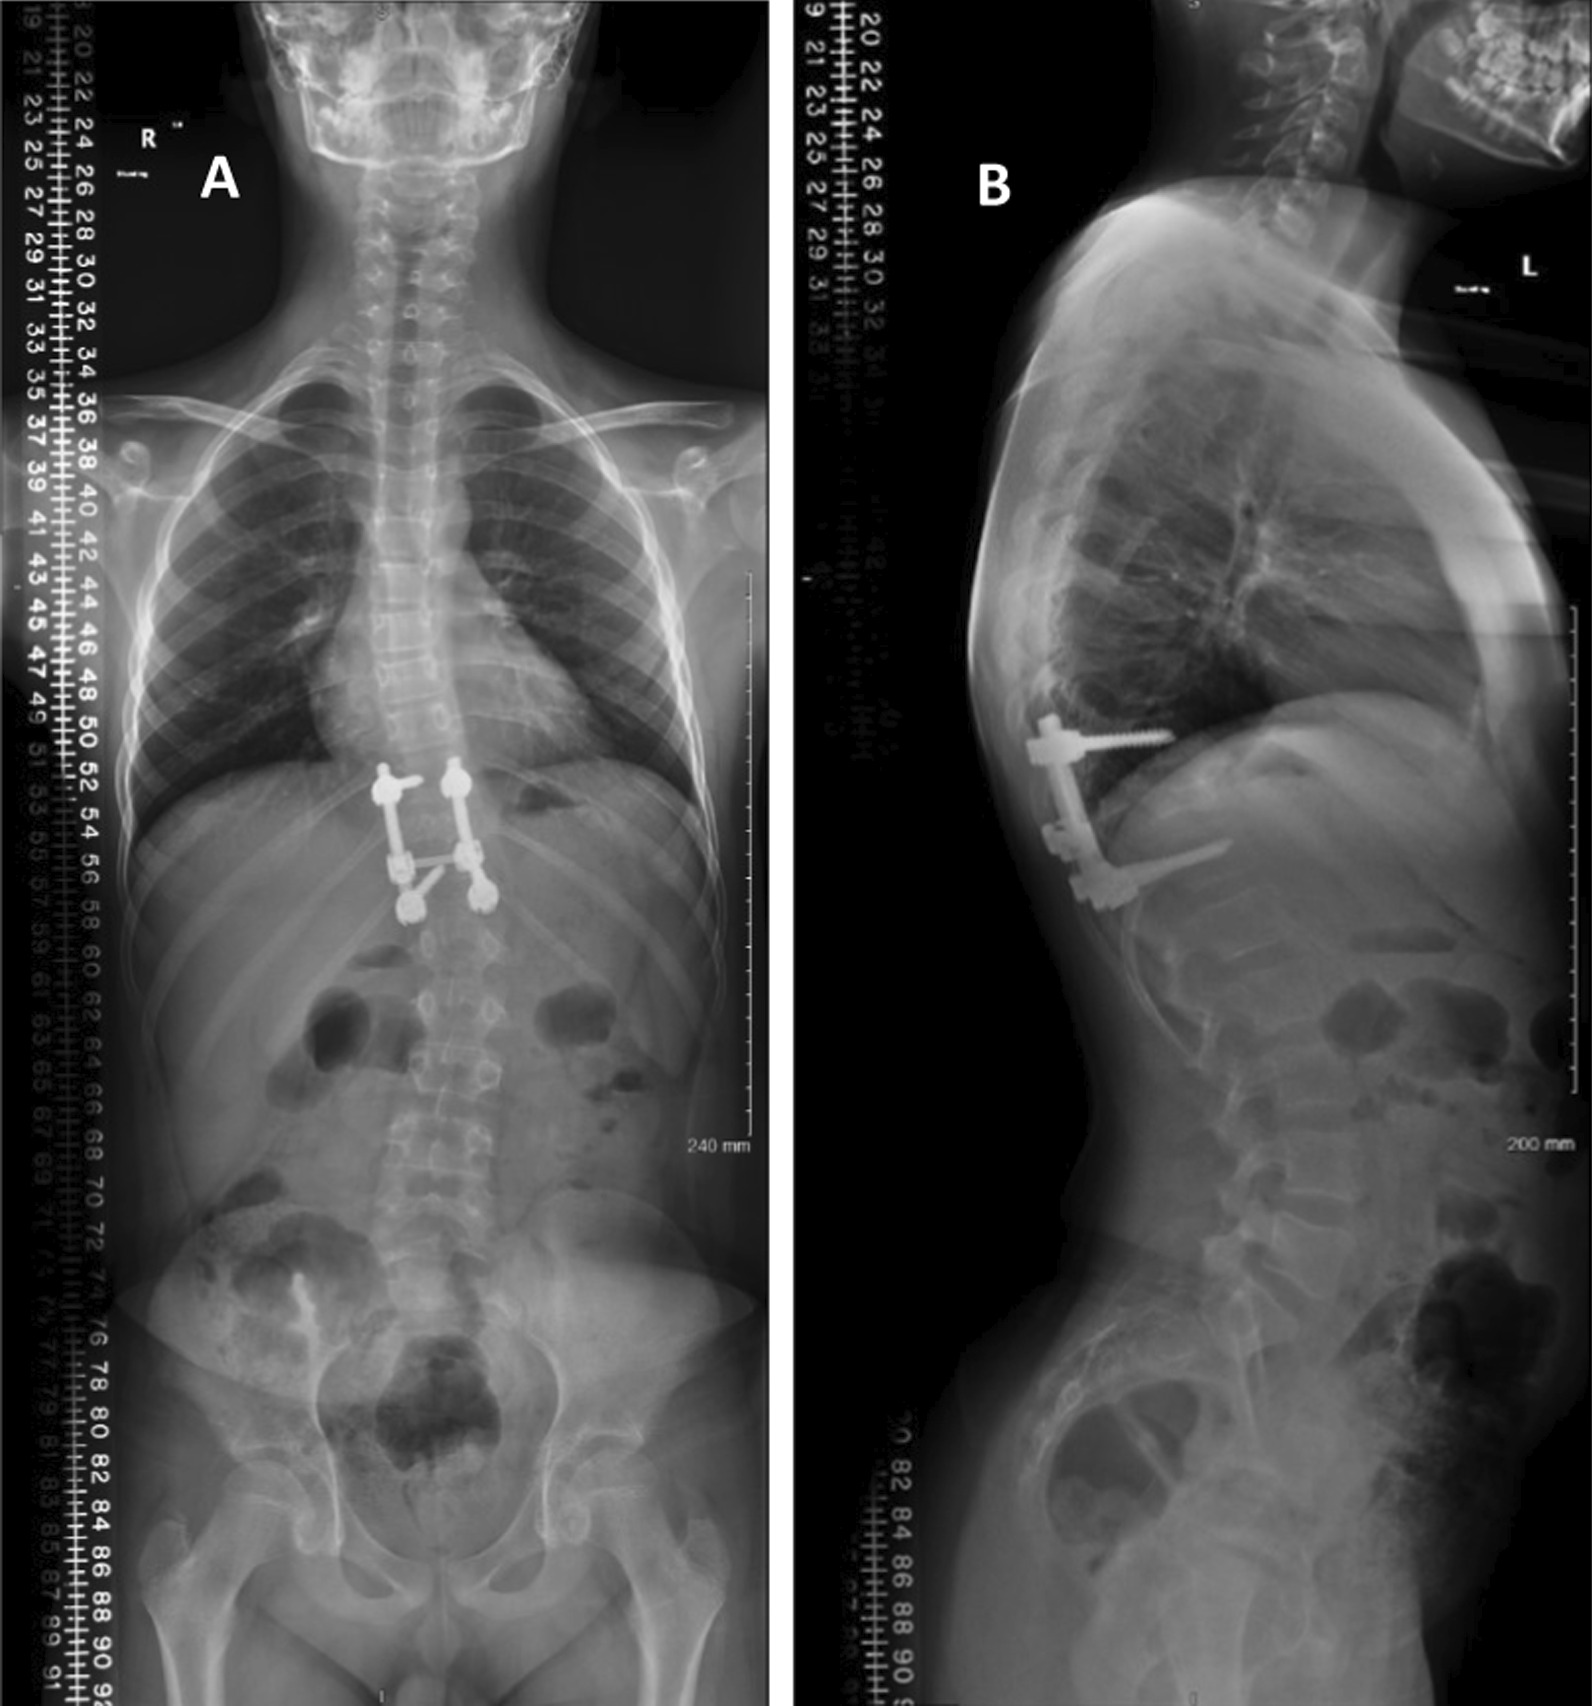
A, B Scoliosis X-ray A AP, and B lateral showing posterior spinal instrumentation from T10 to T12 with an open triradiate cartilage Reiser 0 along with left scoliotic deformity with Cobb angle of 24
